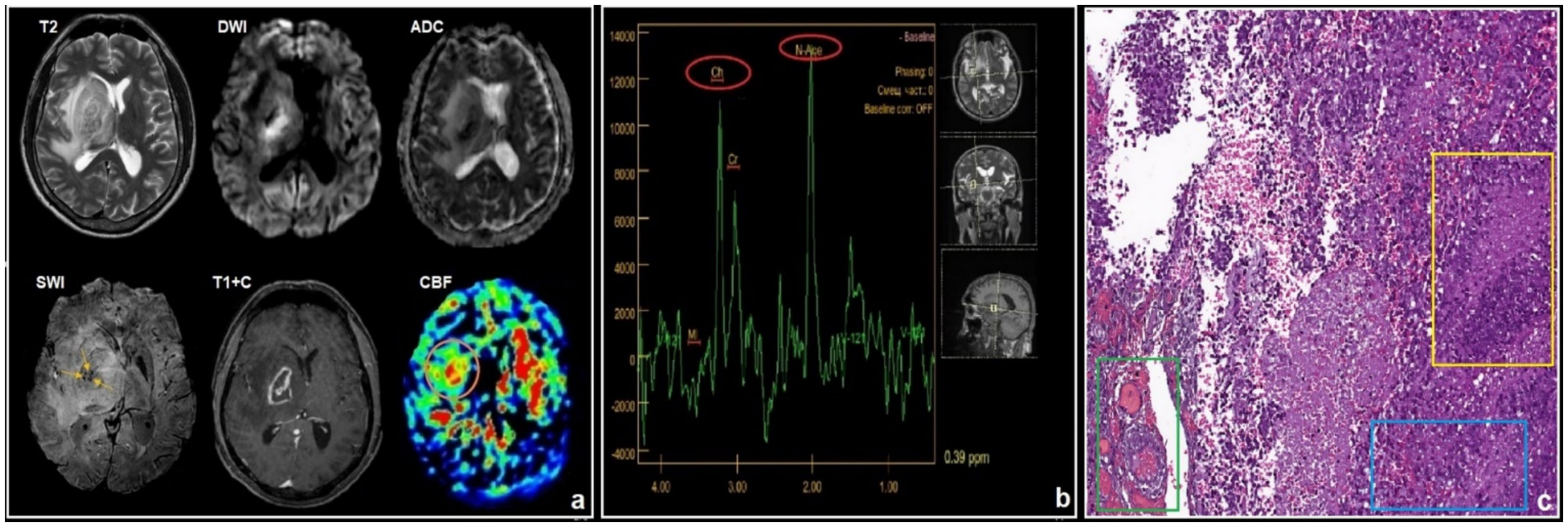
A 41-year-old HIV-positive male, with glioblastoma with acute complaints of weakness in the left extremities and disorientation. The CD4^+^ lymphocyte level count 180 cells/μL. The titer of antibodies to *T. gondii* was 1:400: (a) conventional MRI, (b)
